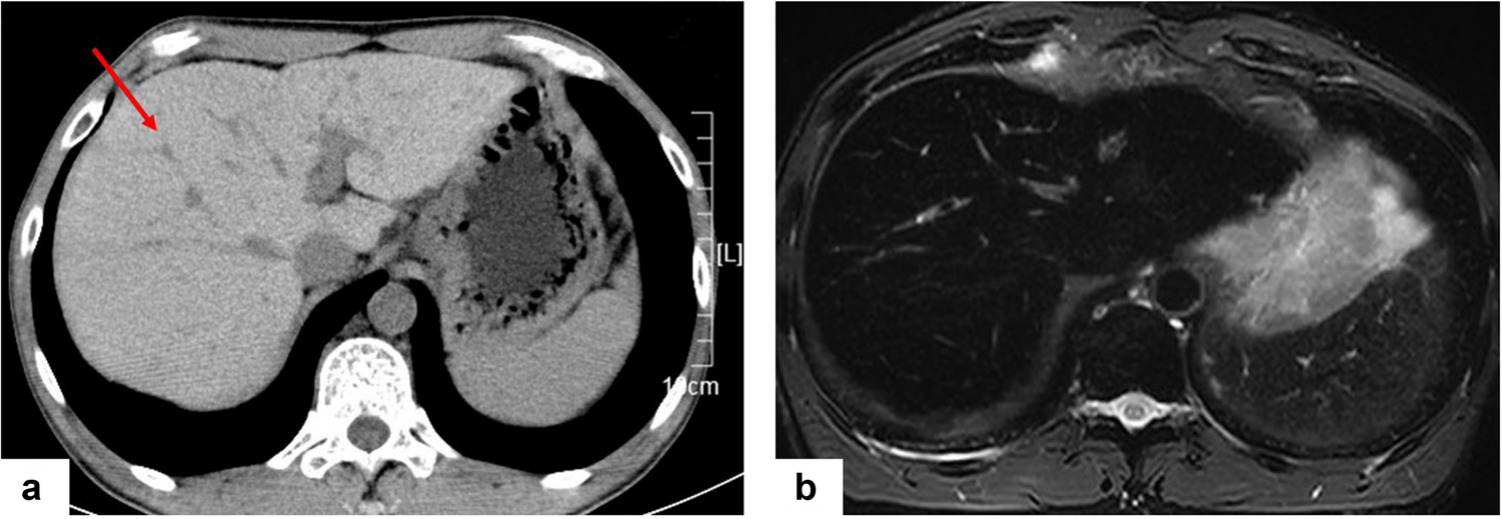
Imaging features of patients. (a) The density of the liver parenchyma increases diffusively in the CT portal vein stage, with a CT value of approximately 96 HU. Liver tissue sampling points (arrows). (b) In the magnetic resonance imaging T2 stage, the signals of the liver and spleen were low.
